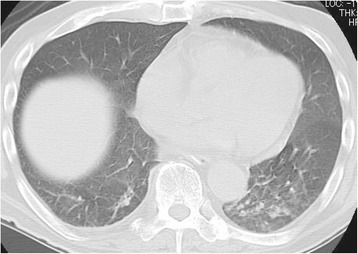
Computed tomography image of nivolumab-induced pneumonitis in Case 1. On the 17th day of nivolumab treatment, non-segmental ground-glass opacities developed at lower lobes of the bilateral lungs
